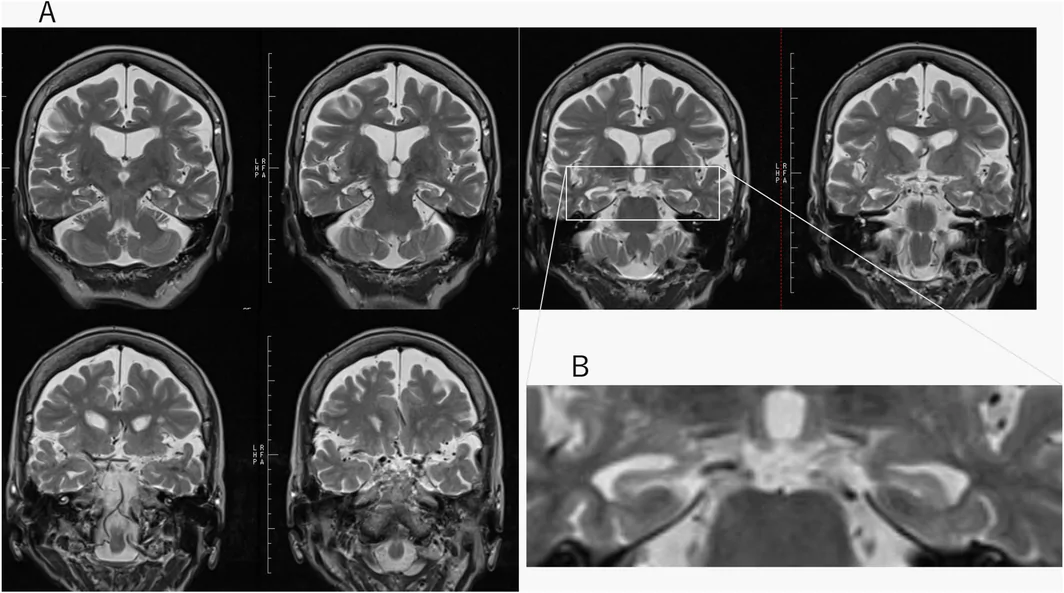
Shows a coronal T2‐weighted image. Mild generalized atrophy is detected (A). There is bilateral medial temporal atrophy including hippocampi and amygdalae, with a slight right‐sided predominance, and blurring of the layered structure of the hippocampus is noted (B). Similar findings were also observed on an MRI taken 20 years ago.
